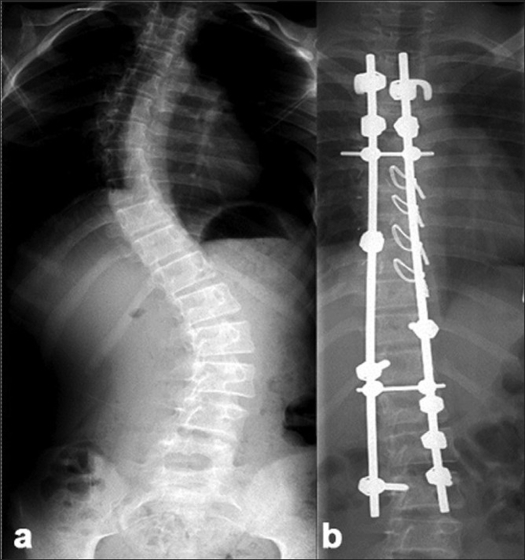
(a) Preoperative X-ray of dorsolumbar and sacral spine (anteroposterior view) of a 12 year old female with Lenke Type1C curve. (b) Post-operative radiograph depicting hybrid constructs with screws at the bottom and hook-claws at the top and sublaminar wires at the concave apex, resulting in a good correction
